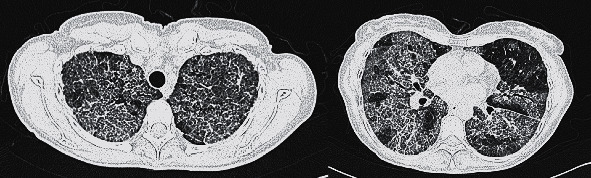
CT scan of the patient during admission showing extensive pulmonary involvement with a crazy-paving pattern (septal thickening and ground-glass opacity) in a patchy distribution throughout the lobes.
